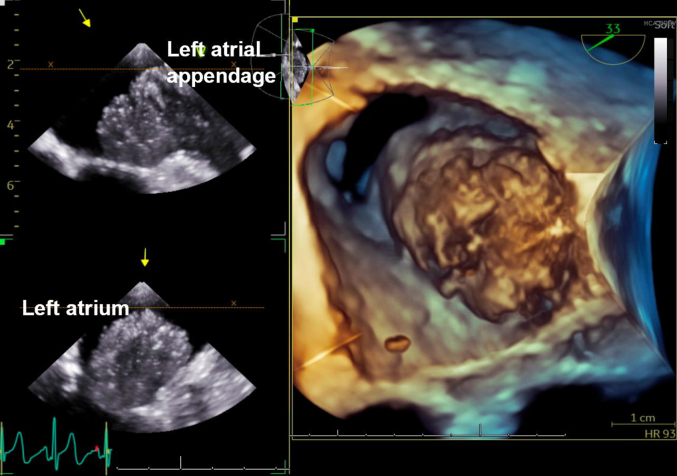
Transesophageal Echocardiography 3-Dimensional Image Showing a Large Mass Originating From the Left Atrial Appendage Without Crossing the Mitral Valve
